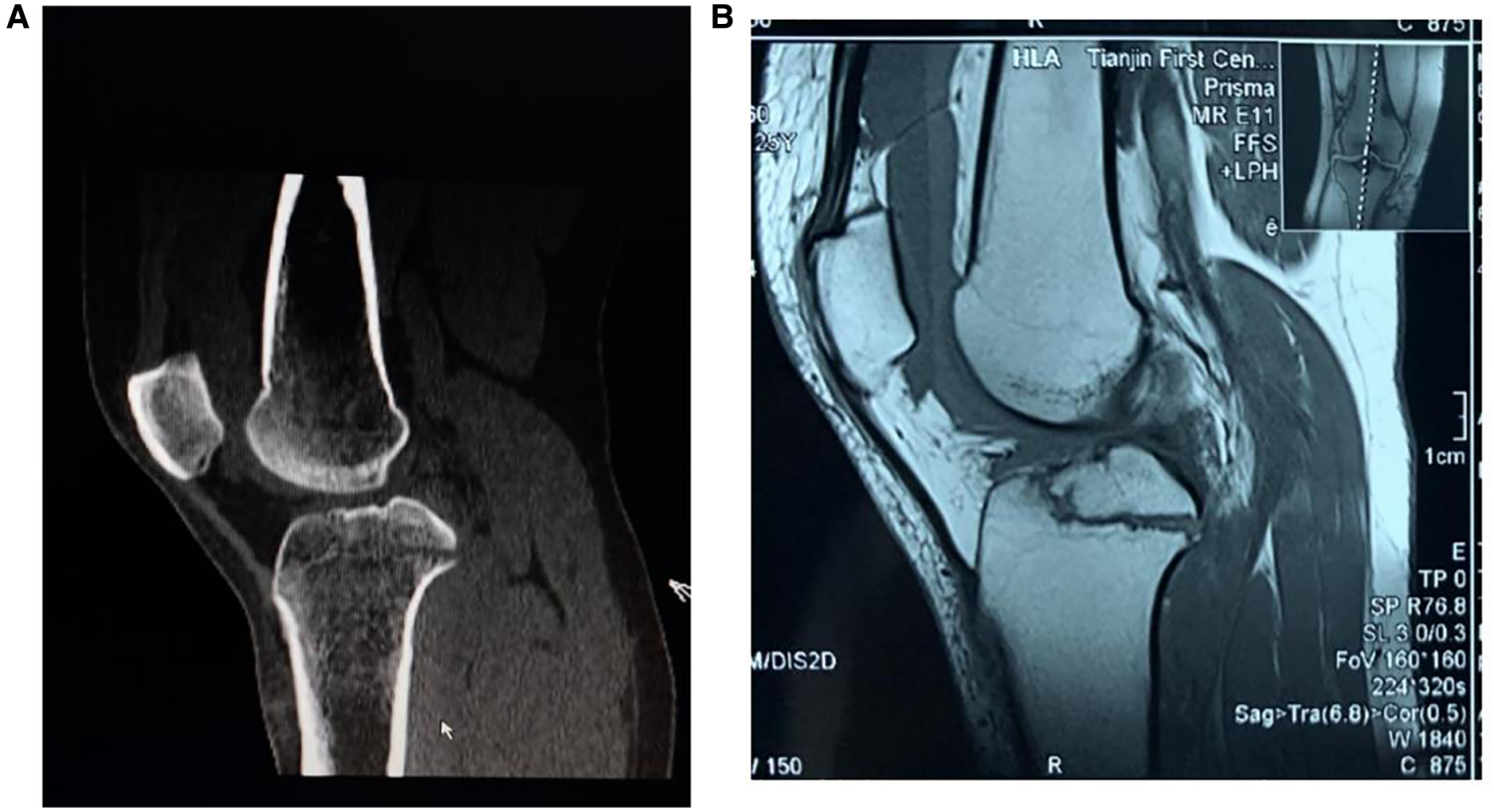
Preoperative radiograph of posterior cruciate ligament (PCL) avulsion fracture. (A) CT scanning showed the posterior cruciate ligament avulsion fracture. (B) MRI confirmed the fragment size and displacement of the PCL avulsion fracture.
